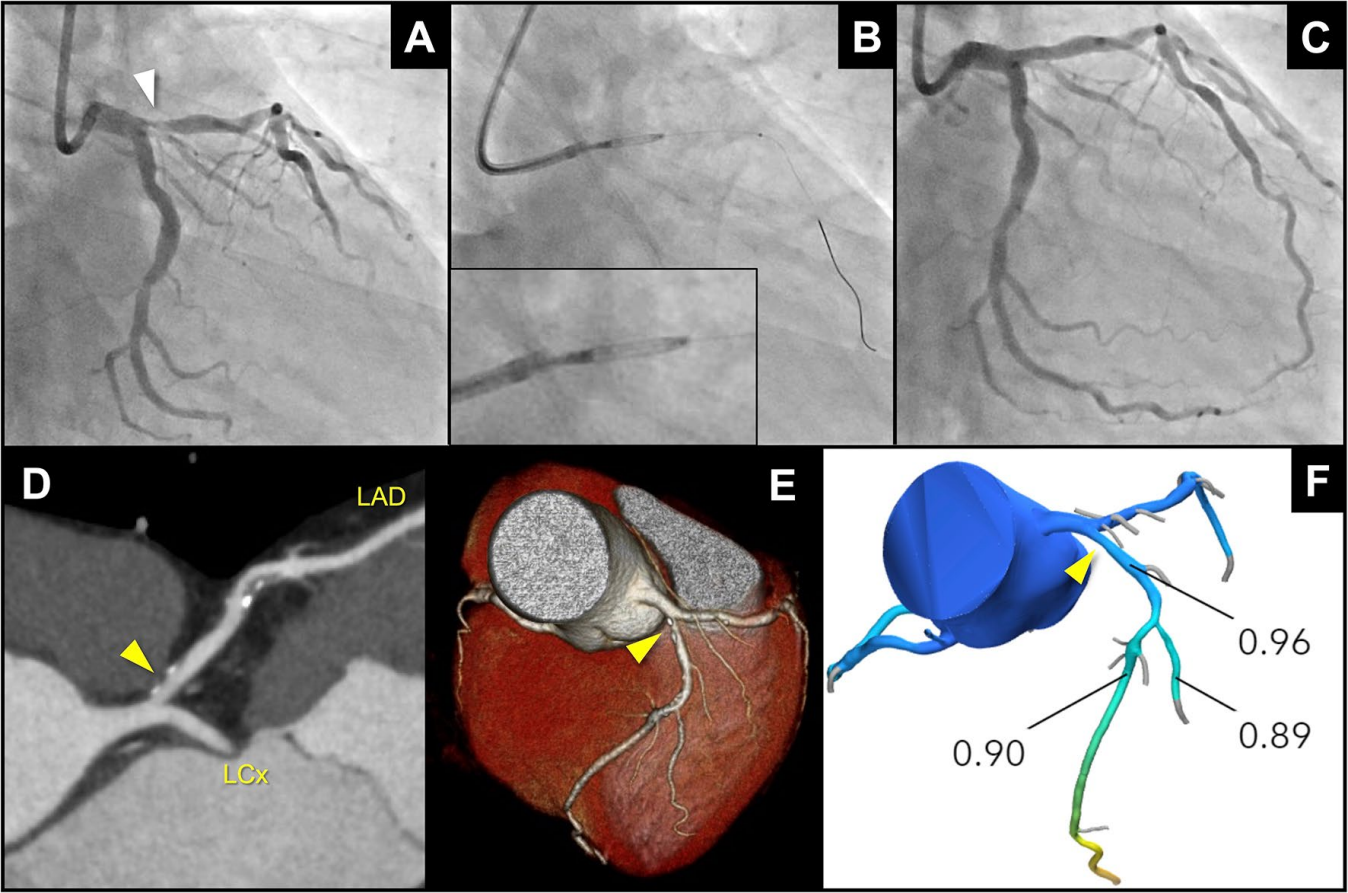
A case treated with DCB-only strategy using directional coronary atherectomy for ostial left anterior descending lesion. A 65-year-old man presenting with chronic coronary syndrome. a Pre-procedural angiogram revealed a focal lesion at ostial left anterior descending (white arrow head). b Plaque was removed by the directional coronary atherectomy (Atherocut®, NIPRO Corp, Tokyo, Japan) followed by a paclitaxel-coated balloon (SeQuent Please Neo®, B. Braun, Melsungen, Germany). c Post-procedural angiographic result was fine. The patient was asymptomatic and scheduled follow-up was planned by the coronary computed tomography (CT) angiography at 12 months after the procedure. The maximum intensity projection (d), volume rendering (e), and CT-derived fractional flow reserve (FFRCT) (f) showed excellent results without any evidence of restenosis (yellow arrow heads). This case highlights an advantage of the “stentless” or “leave nothing behind” strategy in non-invasive anatomical and functional assessment by CT scan at the clinical follow-up
